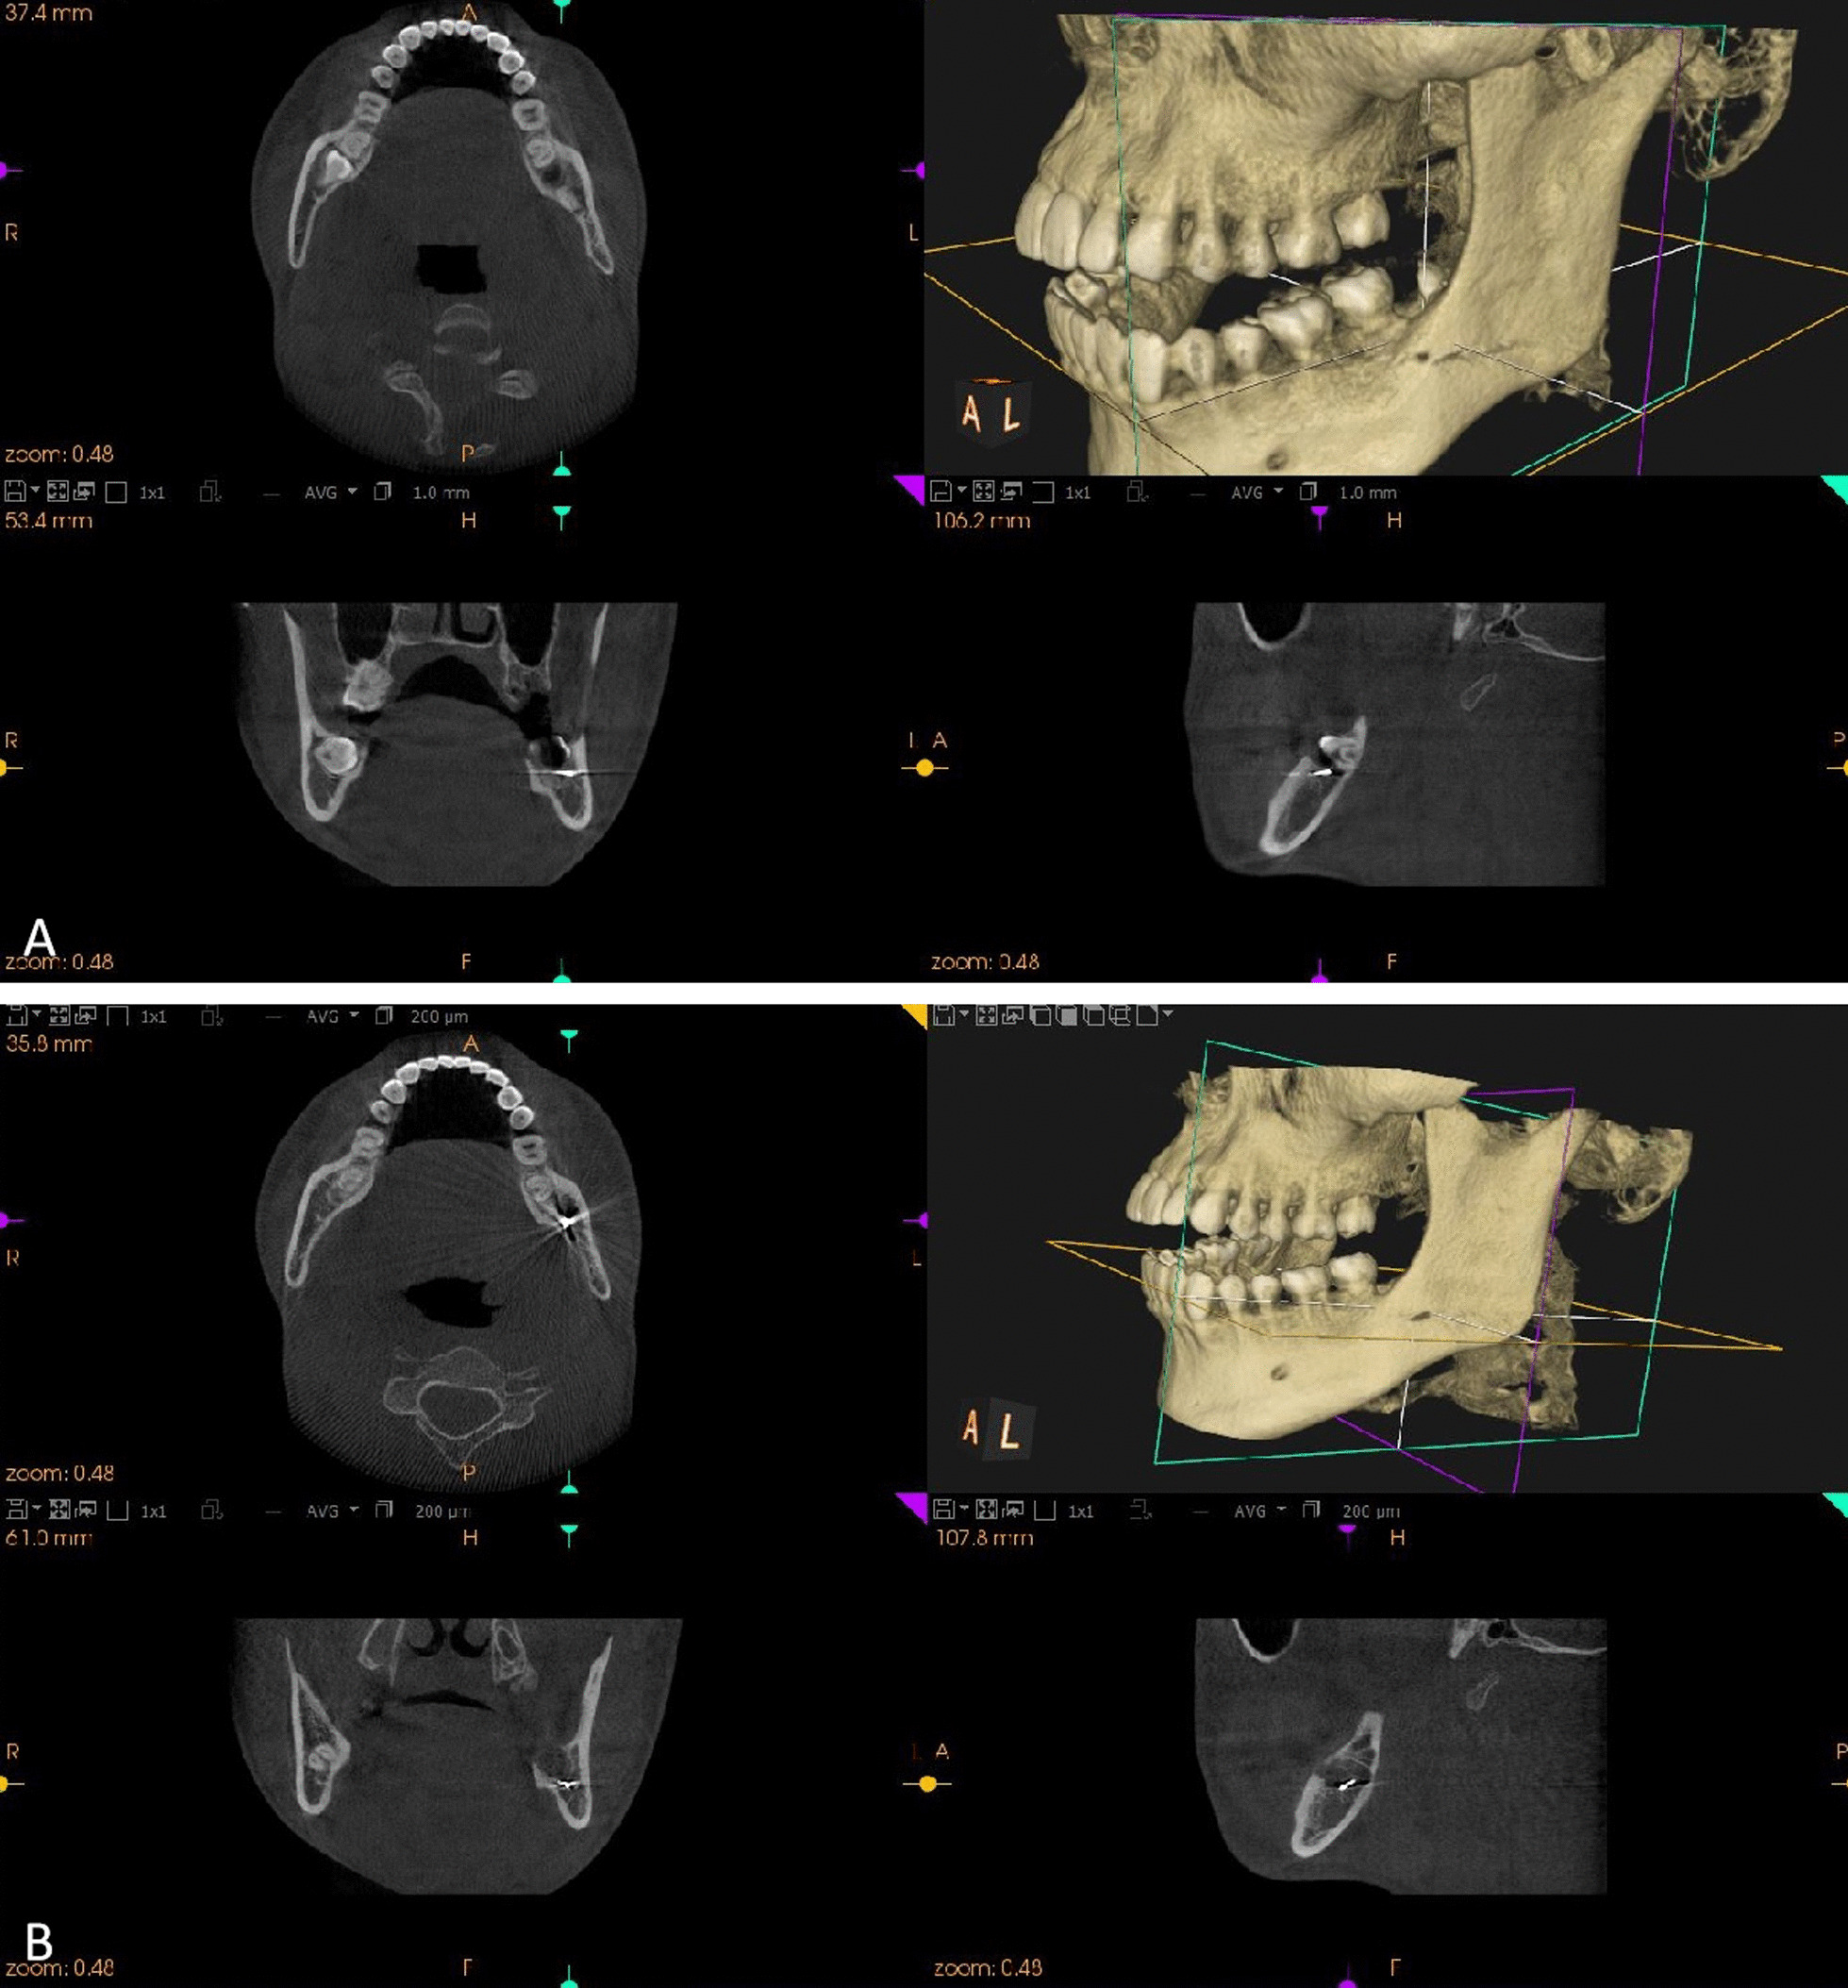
Case 3* A The breakage of the bur during crown sectioning. CBCT immediately showed the 3D position of the bur fragment. B CBCT showing the 3D position of the bur fragment 5 days after tooth extraction
